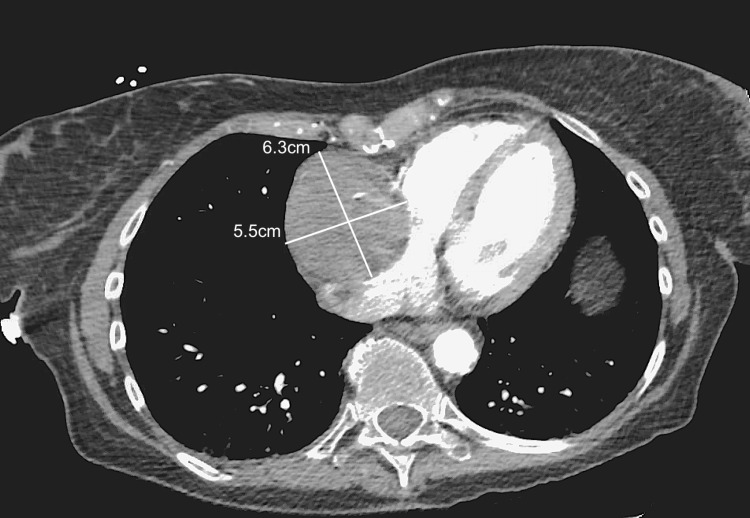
CT chest demonstrating a 6.3cm x 5.5cm x 7cm aneurysmal sac seen abutting the right heart wall with external compression on the right atrium.
